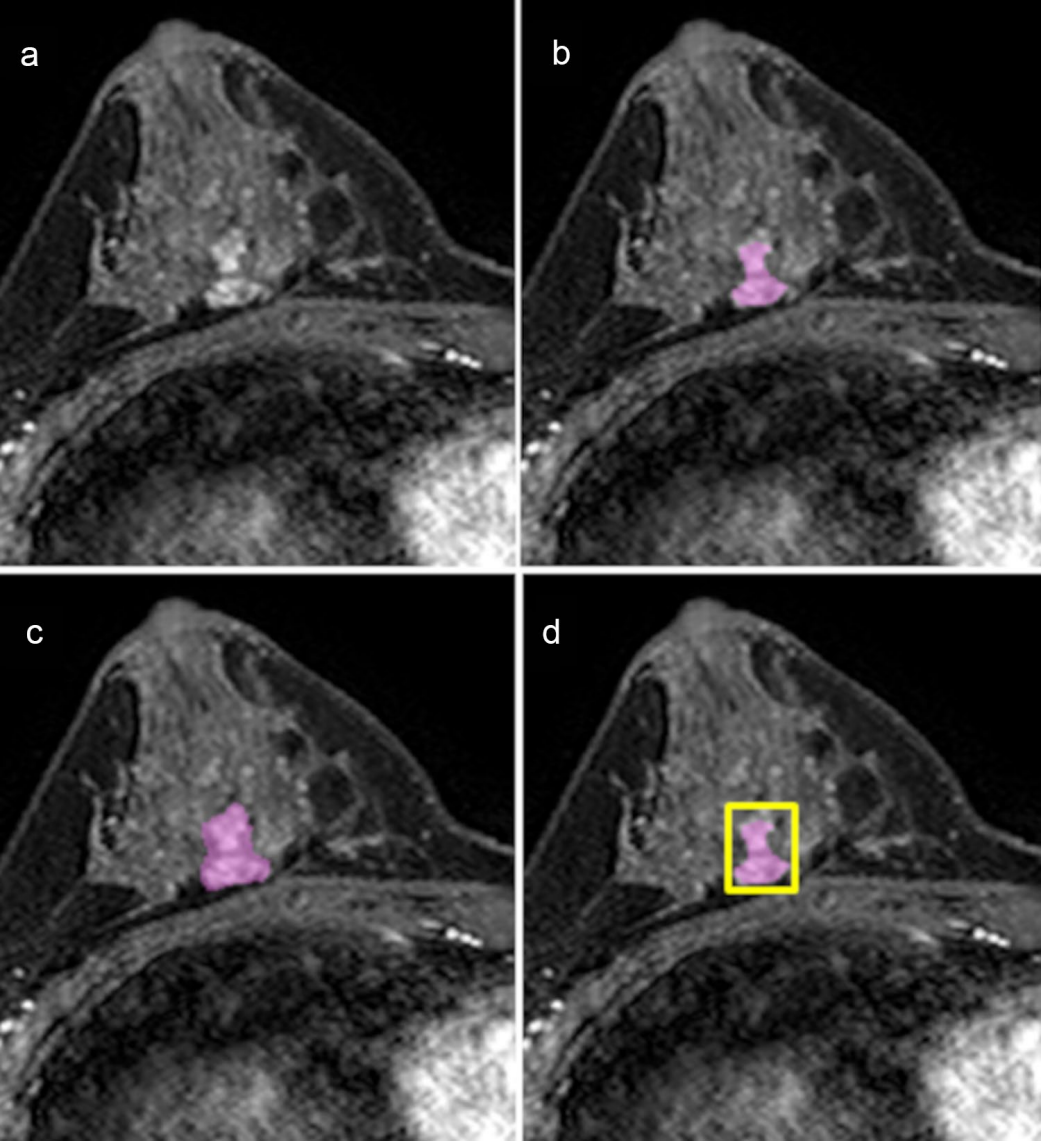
A 39-year-old patient with benign fibrocystic change. a Axial early-phase dynamic contrast-enhanced MRI demonstrates non-mass enhancement in the right breast. Reader 1 (highly experienced radiologist) assessed the lesion with focal distribution and clumped internal enhancement, and finally decided on BI-RADS category 4B. Two patterns of the ROI were set by one radiologist: b ROI-1, precise segmentation that contains only the enhancing lesion, and c ROI-2, rough segmentation that covers the whole enhancing region, including the intervenient non-enhancing area. The input bounding boxes for ResNet50 were generated with the entire lesion d for all slices
